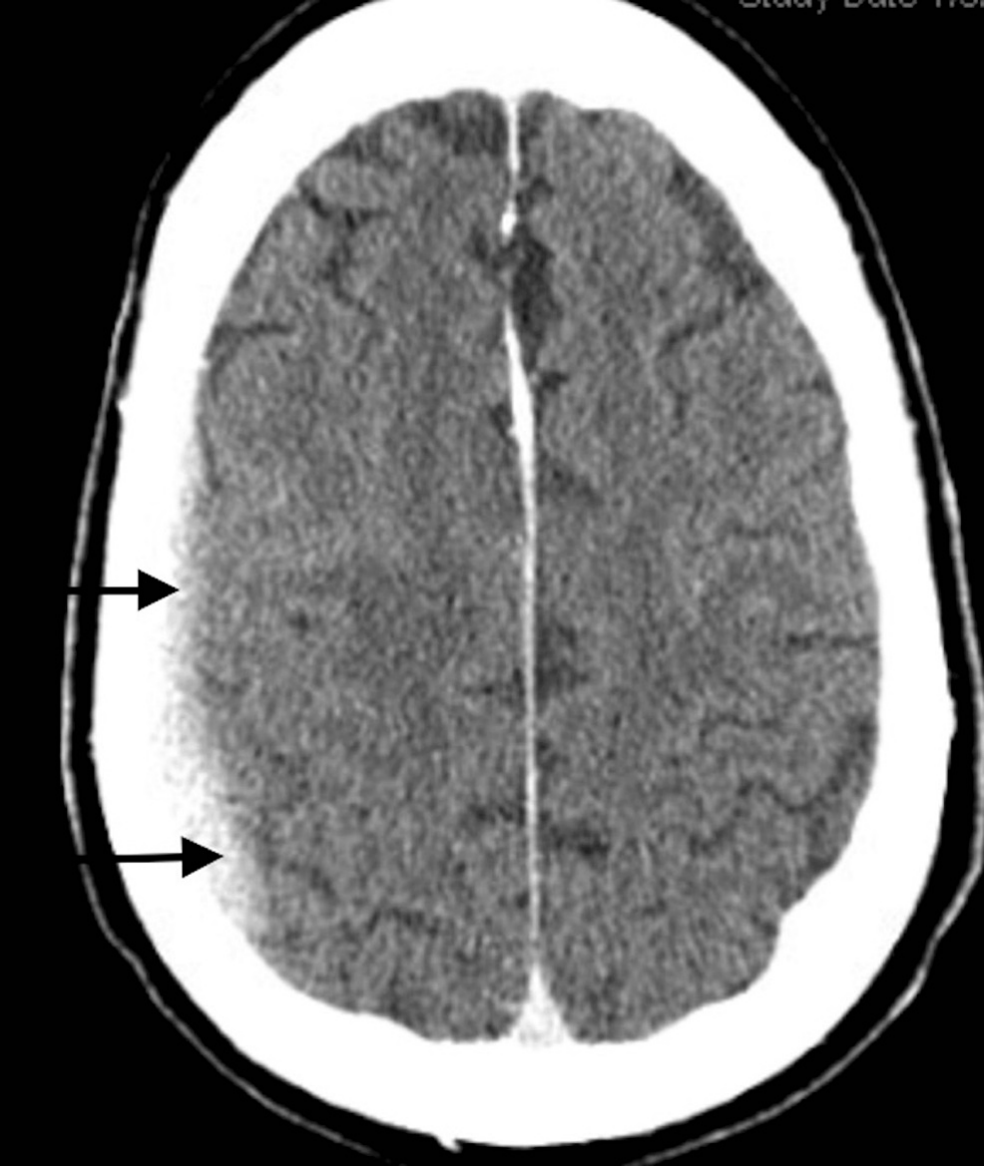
Computed tomography scan of the brain showing a hyperdense collection (see black arrows), which has a contrast effect post-procedure
